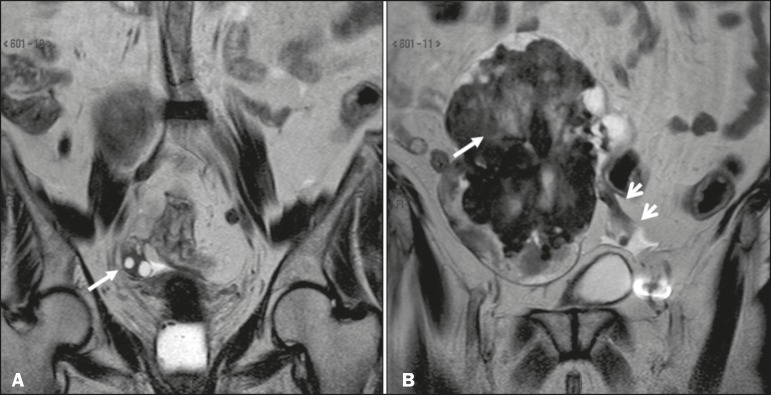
Coronal T2-weighted MRI sequences. A: Right ovary in a normal position with normal volume (arrow). B: Enlarged left ovary shifted to the right due to the presence of hypointense, heterogeneous expansile formation consistent with a fibroma (long arrow). Note also the ectatic vascular pedicle (short arrows).
